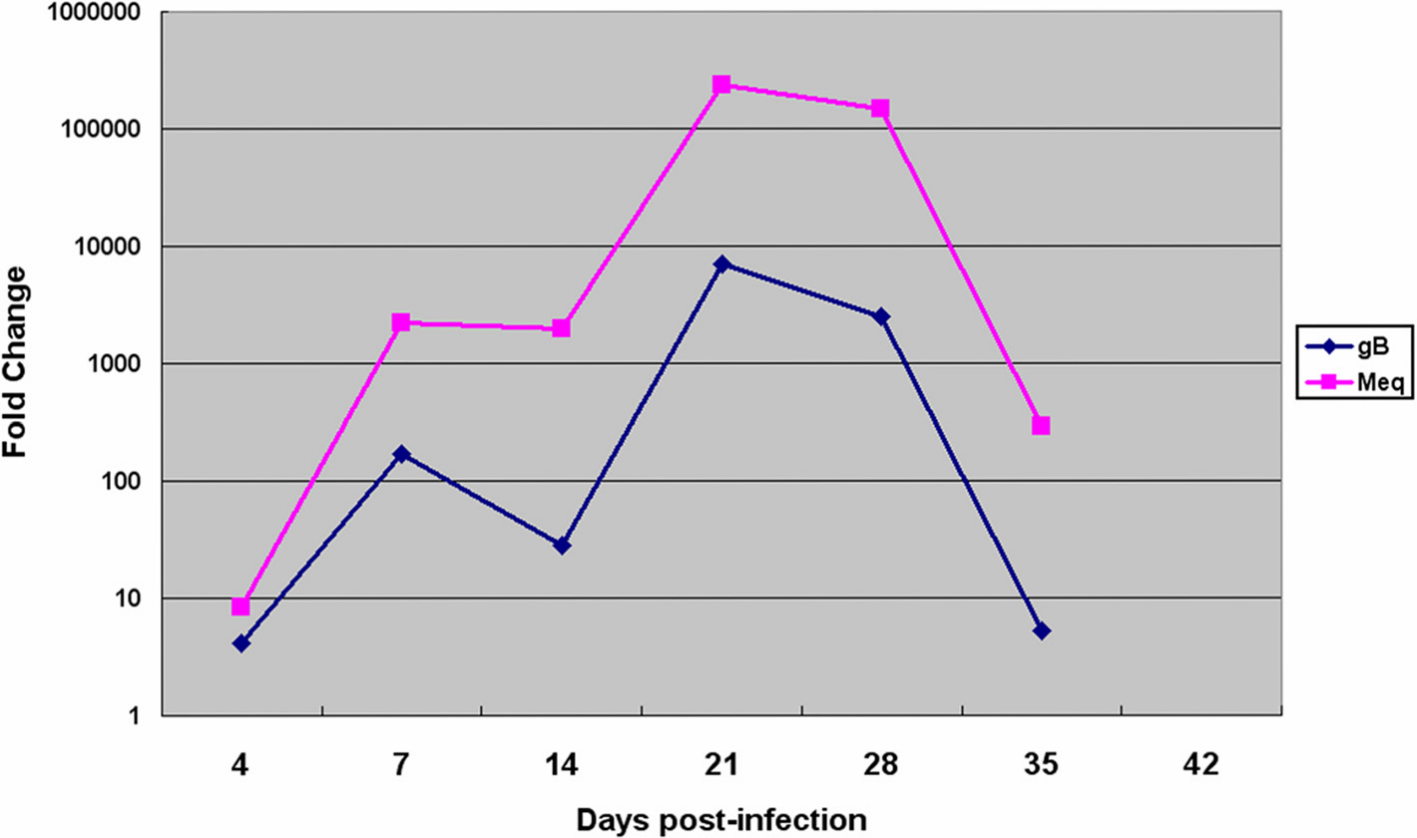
mRNA levels of the MDV transcripts in the chicken thymuses infected with RB1B. The expression levels of Meq and gB mRNA were detected using real-time PCR and calculated using the 2 –^ΔΔ^CT method.
